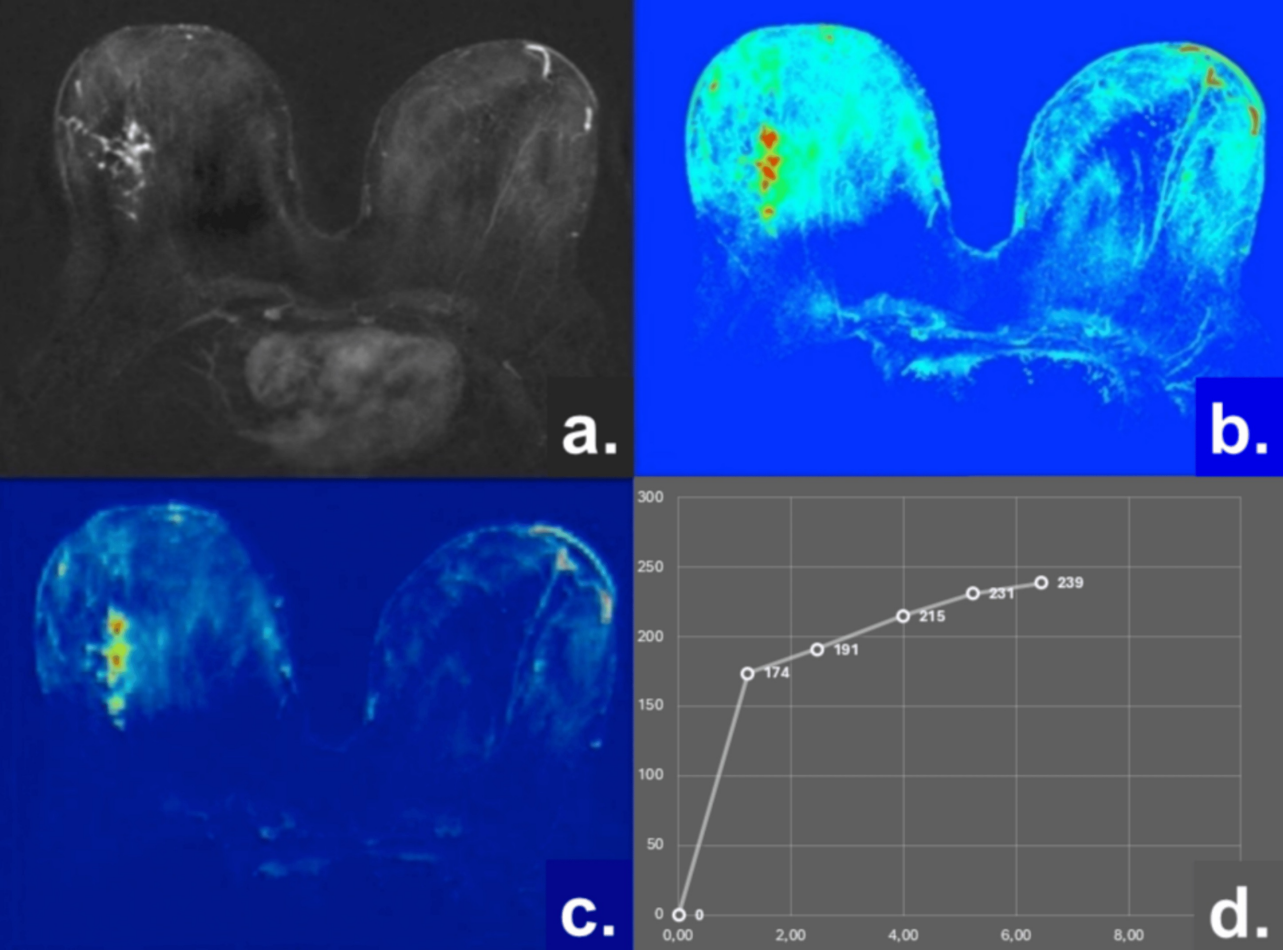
Representative multiparametric breast MRI findings in a patient with ADH (a) Subtraction image demonstrating non-mass enhancement in the right breast; (b) ADC map (1.35 × 10⁻³ mm²/s); (c) PEI = 590.4 ± 20.6; (d) TIC showing a persistent enhancement pattern (TIC Type 1). Abbreviations: ADH, atypical ductal hyperplasia; NME, non-mass enhancement; ADC, apparent diffusion coefficient; PEI, positive enhancement integral; TIC, time–intensity curve.
